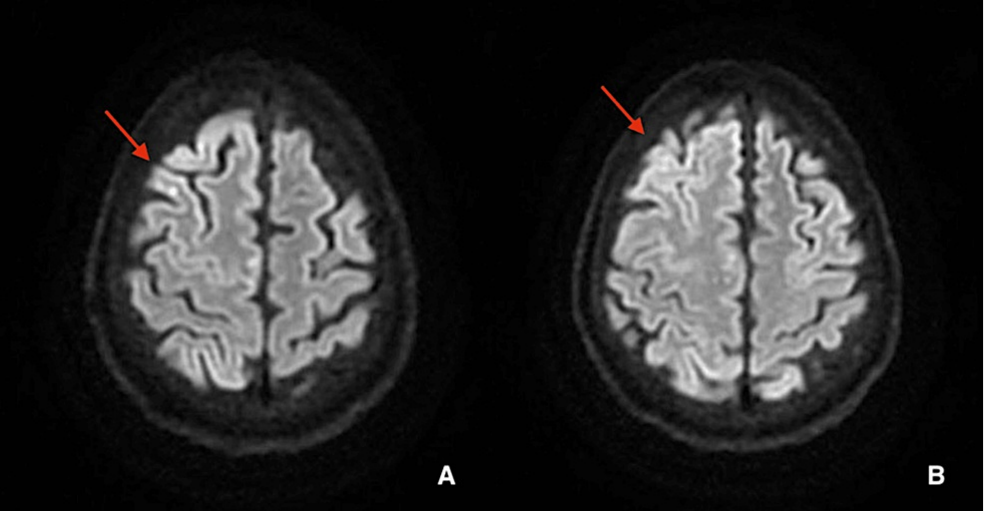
(A and B) Axial brain MRI images revealing right frontal lobe infarcts (red arrows). MRI, magnetic resonance imaging
